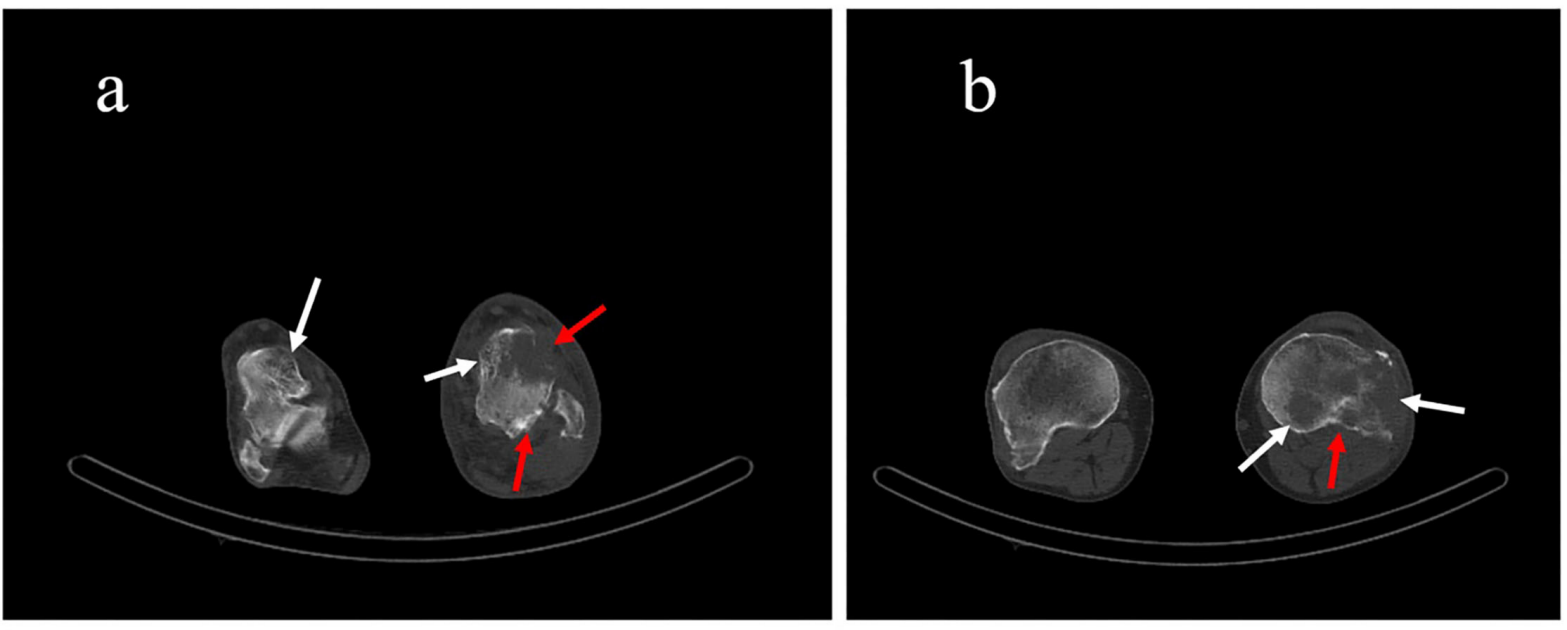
(a) Axial and (b) sagittal computed tomography scan planes, showing irregular accumulation of contrast in the penis without distant metastases (white arrows).
